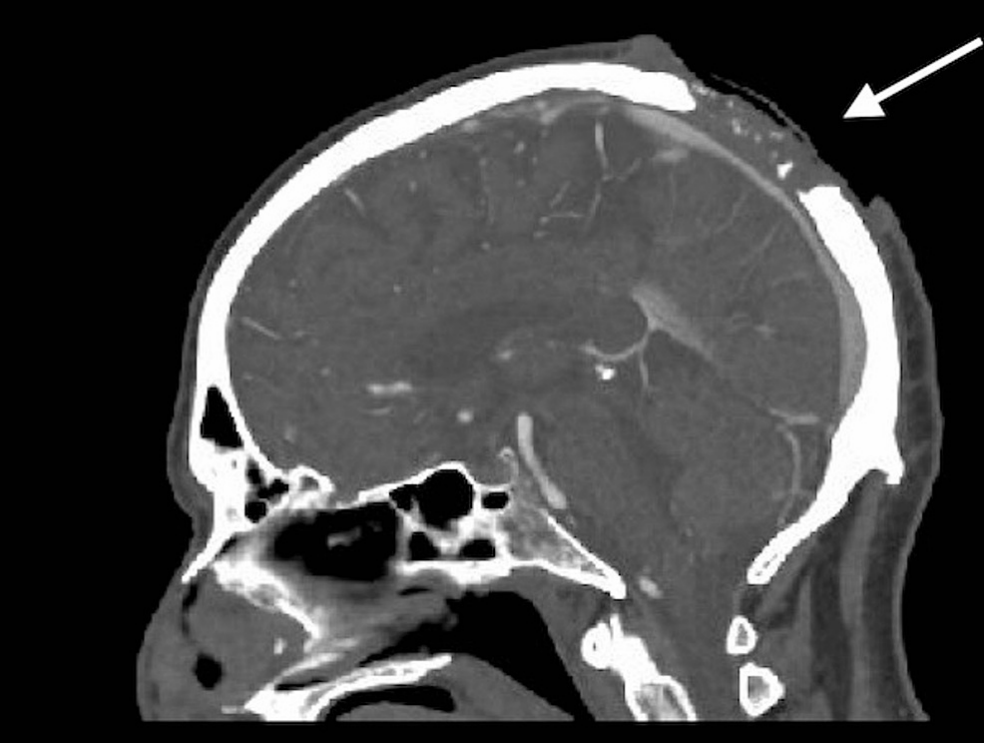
Sagittal images from head CT with contrast demonstrating scalp lesion invading the skull
